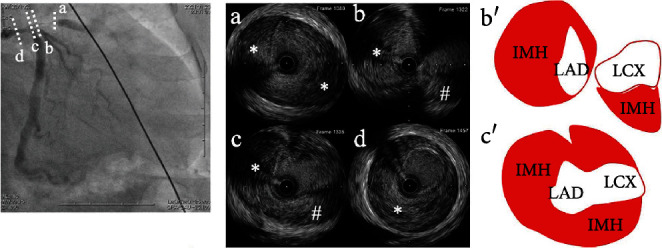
IVUS images of the left main coronary artery to left anterior descending artery and scheme of IVUS images. (a) IVUS image of the left anterior descending artery showing intramural hematoma (asterisk) compressing the true lumen. (b, b′) IVUS images of left anterior descending artery ostium showing intramural hematoma (asterisk). The left circumflex artery and intramural hematoma (sharp) extending to the left circumflex artery can also be observed. (c, c′) IVUS image of left main coronary artery bifurcation showing intramural hematoma extension to the left anterior descending artery (asterisk) and left circumflex artery (sharp). (d) IVUS image of left main coronary artery showing intramural hematoma (asterisk) compressing the true lumen. IVUS: intravascular ultrasound; LAD: left anterior descending artery; LCX: left circumflex artery; IMH: intramural hematoma.
